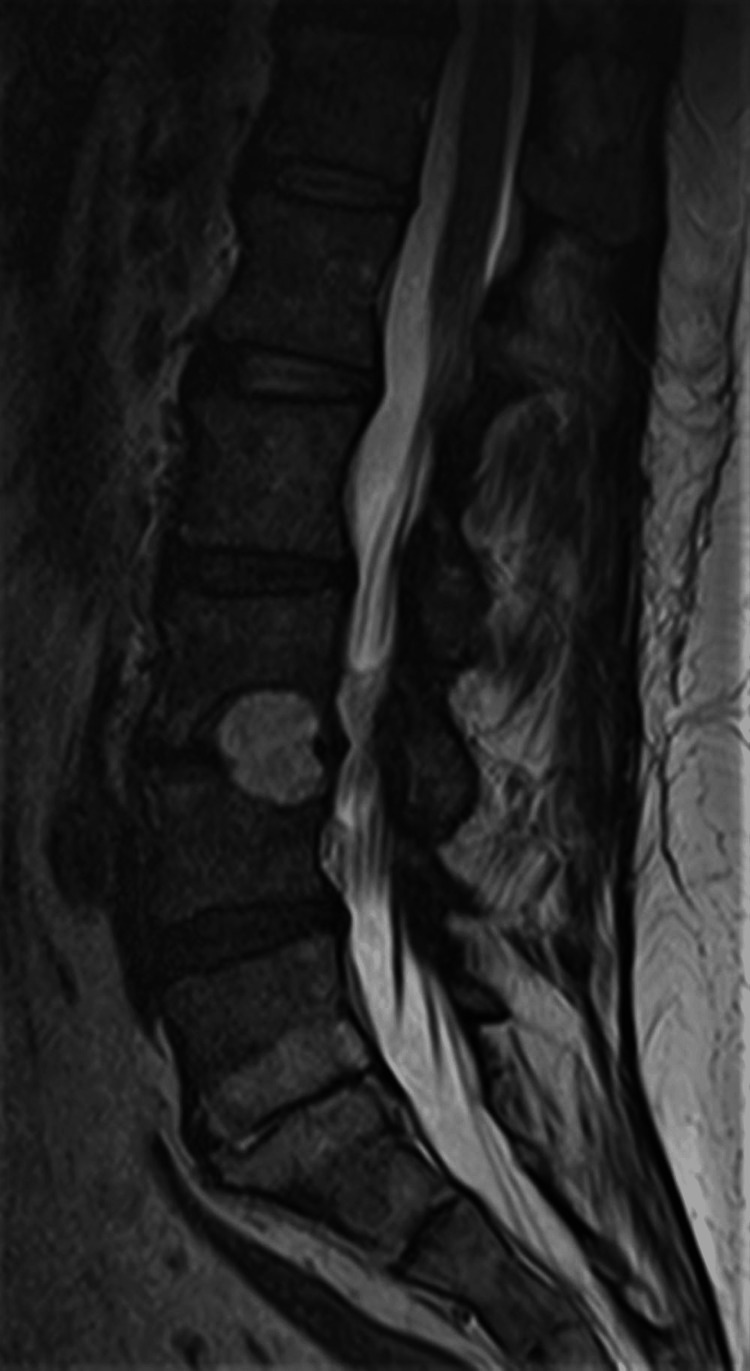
Last control MRI, 11 months after the beginning of the treatment (sagittal view)
